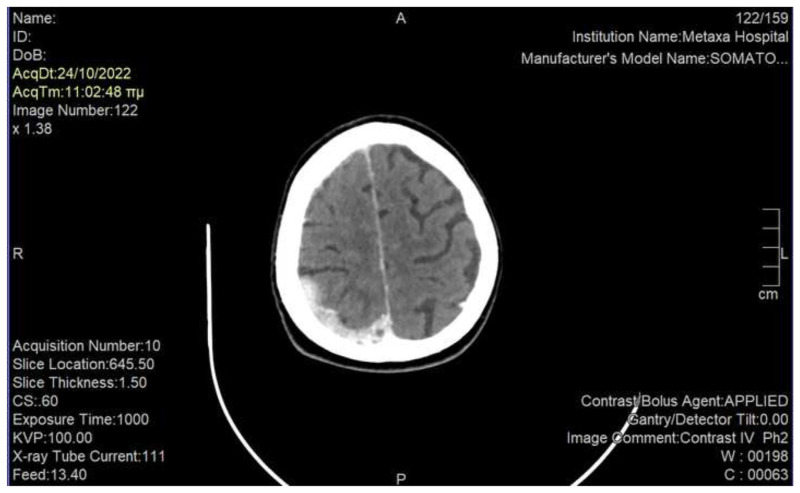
Brain CT showing enhanced leptomeningeal uptake in the right parieto-occipital lobe.
